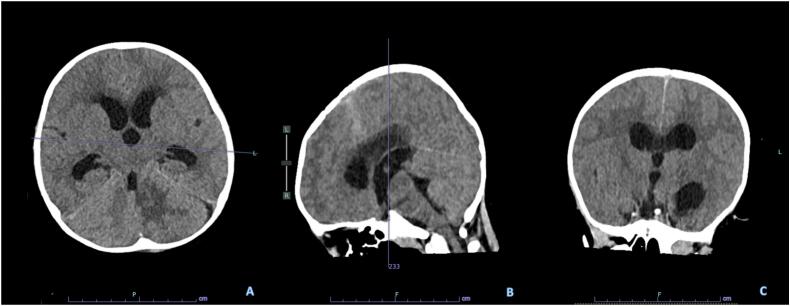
Head CT Scan with without contrast, revealing a complete removal of the tuberculoma mass, there's no obstruction of the ventricular system. As there are dilatation of all the cerebral ventricles, suspicion of tuberculous meningoencephalitis was raised.
